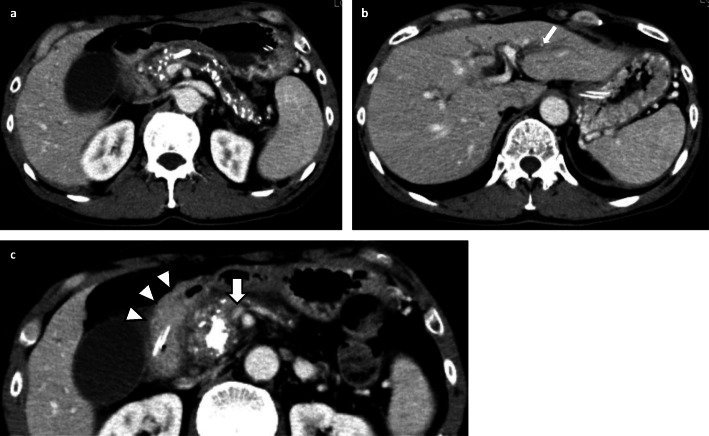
Findings of enhanced CT. Diffuse pancreatic stones and dilation of the pancreatic duct were observed (a). CT also showed slight dilation of the intrahepatic bile ducts (b), thickening of the duodenum wall (c, white arrow head), and stenosis of the superior mesenteric vein (c, white thick arrow)
